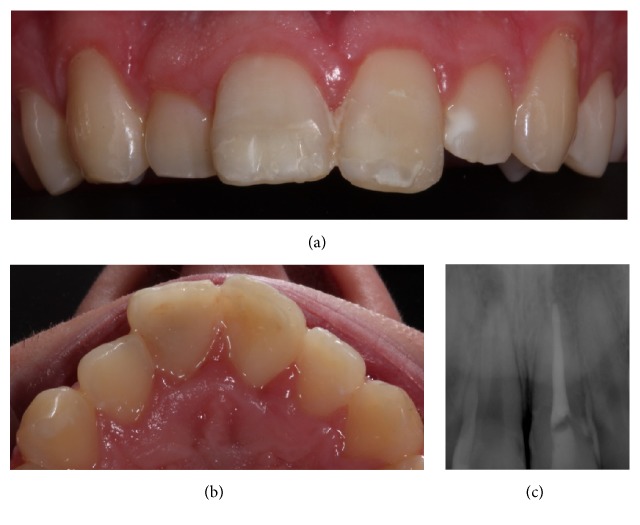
(a) Clinical aspect of buccal view after 1-year follow-up. (b) Clinical aspect of palatal view after 1-year follow-up. (c) The periapical radiograph after 1-year follow-up.
